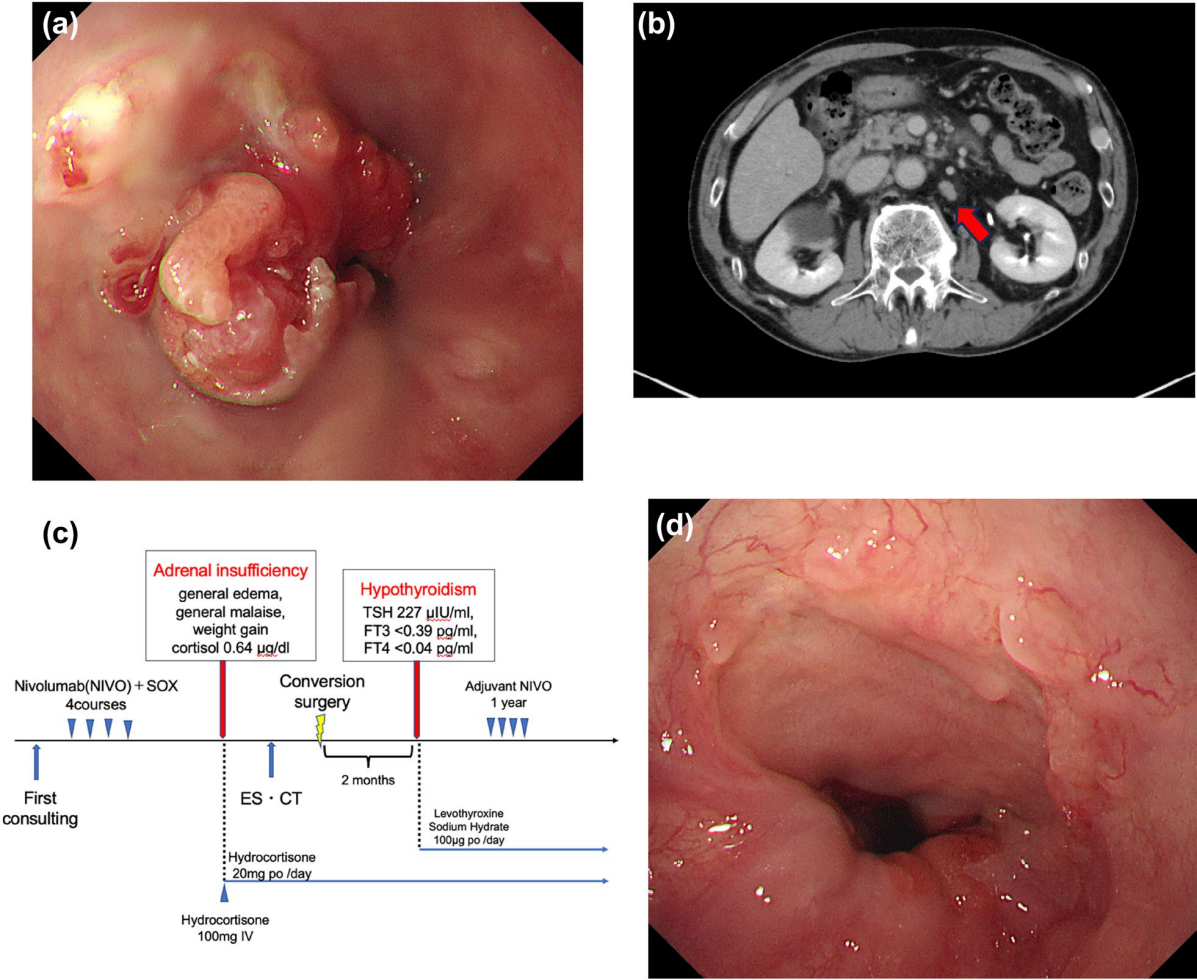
Imaging for Case 1: Initial EGD showing a Type 2 lesion around the EGJ with 3 cm esophageal invasion (a). Enhanced CT demonstrating #16 LNs (b). Treatment course (c). EGD showing marked shrinkage of the primary tumor (d) following induction of ICI-combined chemotherapy. EGD, esophagogastroduodenoscopy; EGJ, esophagogastric junction; CT, computed tomography; LN, lymph node; ICI, immune checkpoint inhibitor
